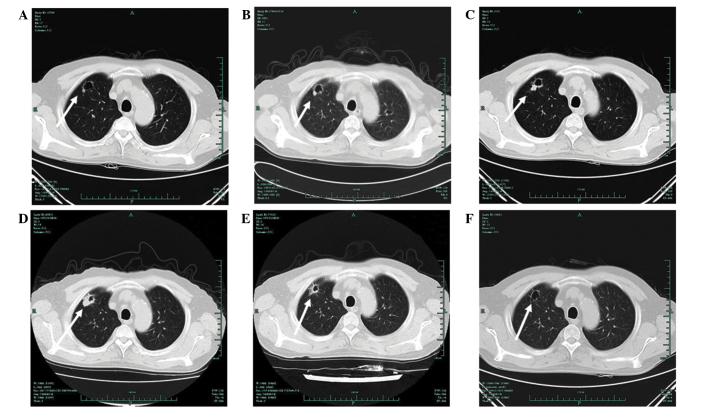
Chest computed tomography (CT) findings showing evidence of a tumor response. Chest CT images showed a tumor response prior to and after the patient received icotinib. (A) The first appearance of a nodule on August 12, 2010, at four months post-kidney transplant. (B) On February 9, 2011, the nodule remained stable as previously. (C) On October 13, 2011, CT imaging showed a marginal increase in the size of the nodule. (D) On February 15, 2012 (prior to treatment with icotinib), the nodule was pathologically diagnosed as adenocarcinoma. (E) Imaging results on March 12, 2012, following one week of treatment with icotinib. (F) Imaging results on August 7, 2012, following five months of treatment with icotinib. The patient met the Response Evaluation Criteria in Solid Tumors (RECIST) for a partial response. The arrows indicate the tumor site.
